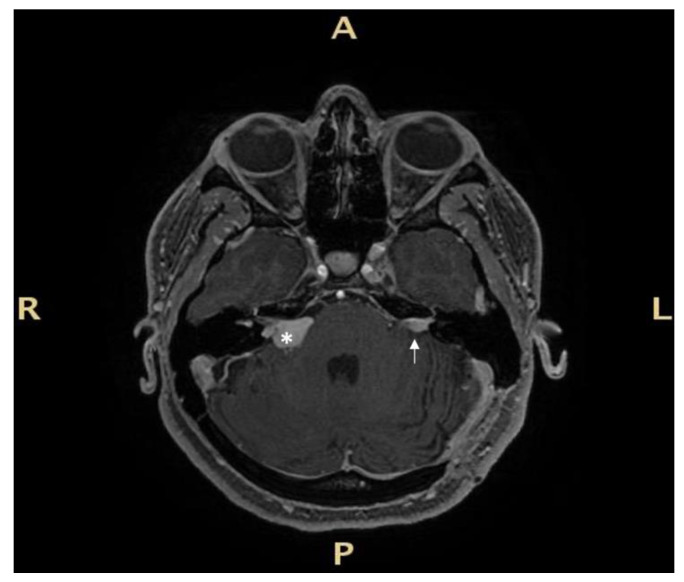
Magnetic resonance Imaging (MRI) before right cochlear implantation: right irradiated and stable tumour with intralabyrinthine component (white asterisk), and left side intrameatal recurrence (white arrow) after surgery via a retrosigmoid approach. R: right, L: left, A: anterior, P: posterior.
